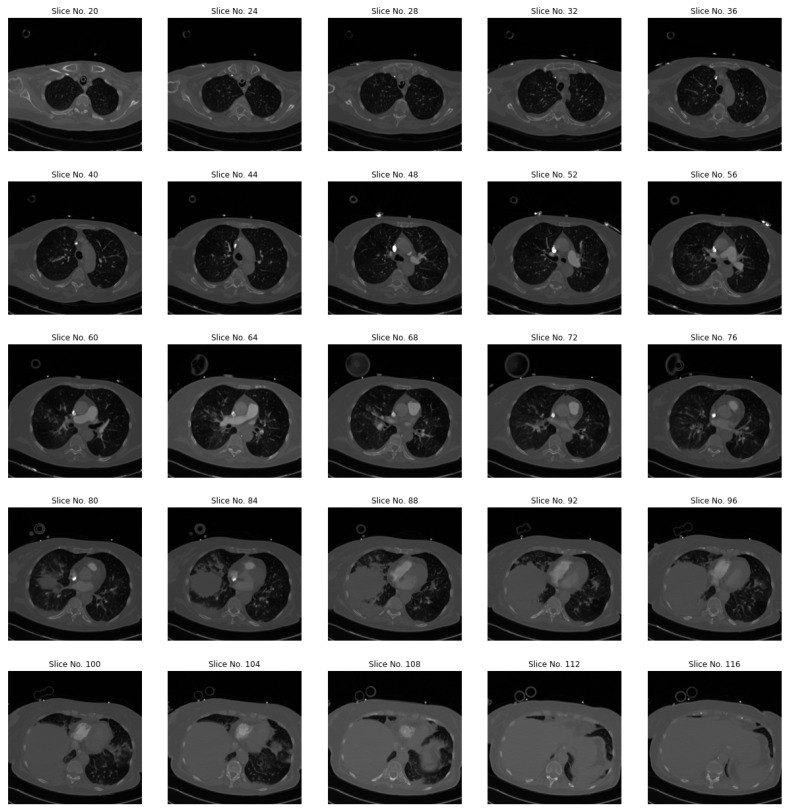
Sample CT images of a randomly chosen subject from the dataset.
